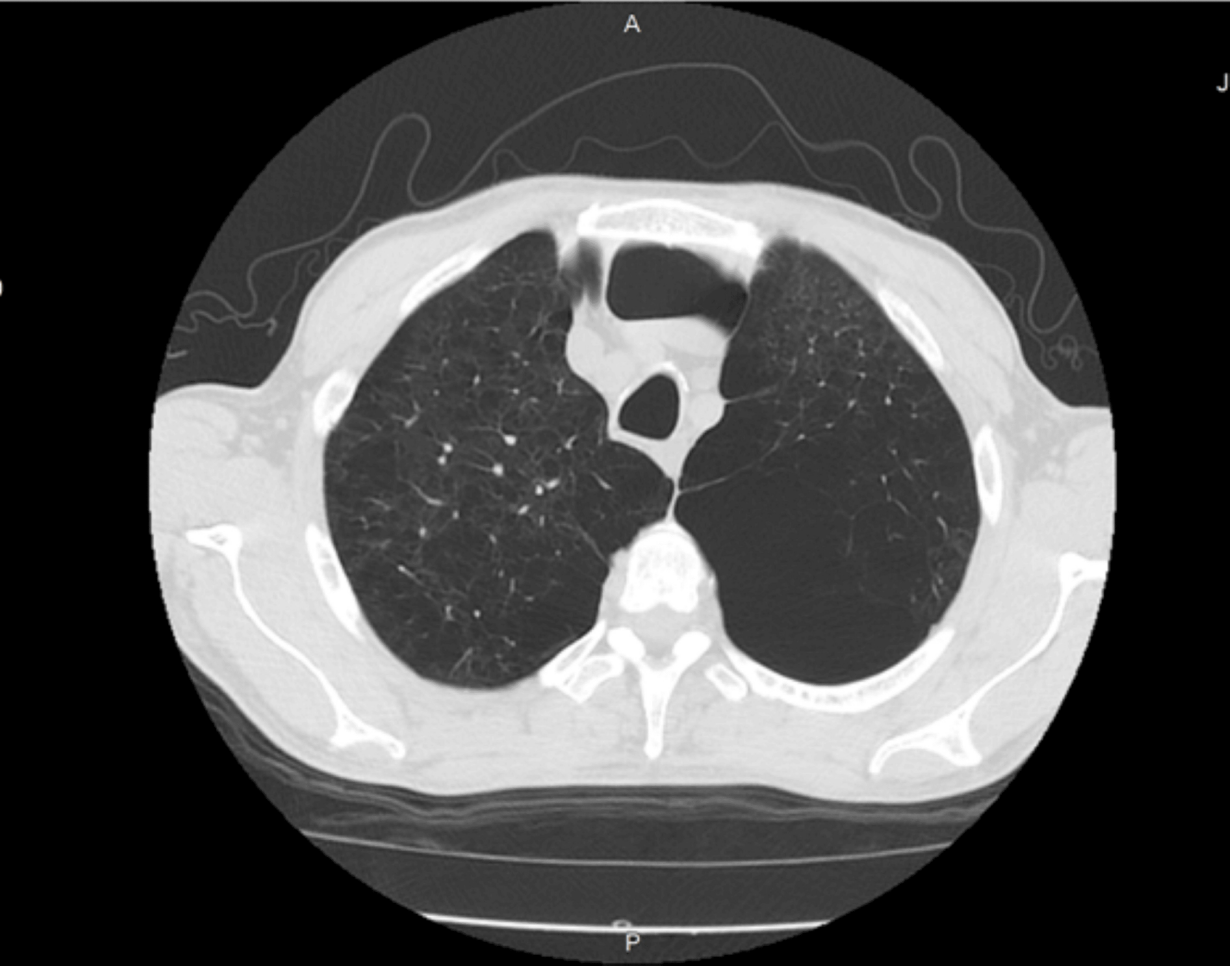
CT scan, at the mid-lung's plane, November 2022
